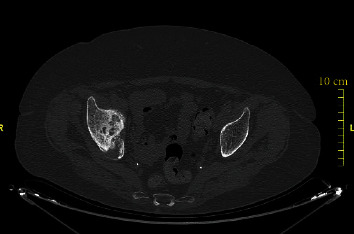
Axial CT-scan view showing the acetabular nonunion on the right.
